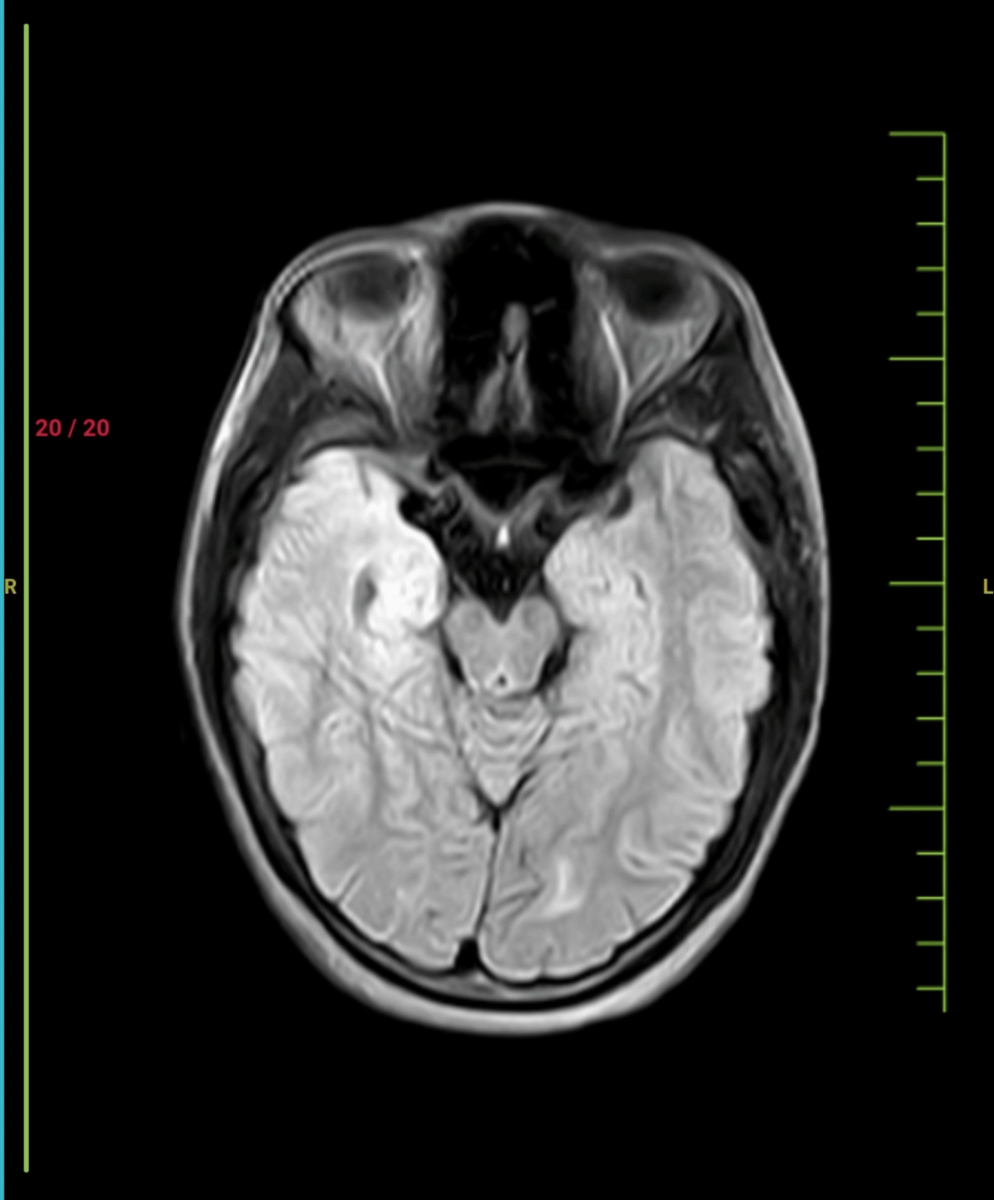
Axial fluid-attenuated inversion recovery sequence of MRI brain of the patient showing no obvious abnormality in the brain parenchyma.
